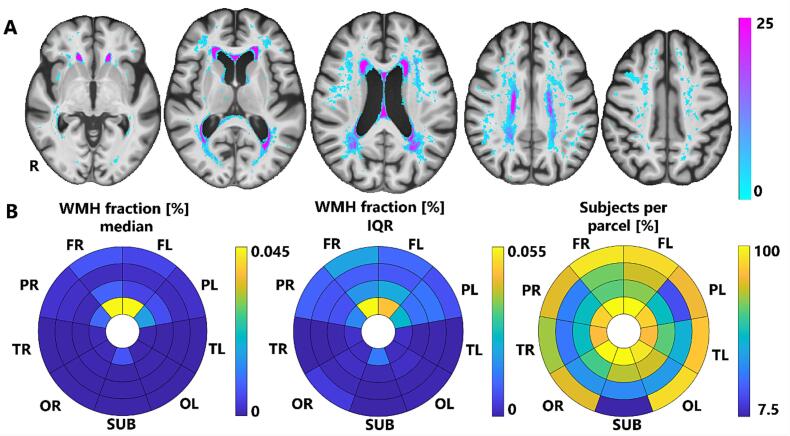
Spatial distribution of WMH. A. Spatial distribution of WMH at 7 T MRI. Fractions of subjects over the entire sample (%, N = 40) are represented. The resulting map is overlaid onto the MIITRA elderly template (axial slices are Z = 2, 13, 24, 34, 43, from left to right). B. WMH fraction distribution (normalized over ICV) indicated by the median (leftmost) and IQR (middle) values, respectively. The rightmost bullseye plot depicts how many subjects (as a fraction of the whole sample, %) showed a WMH lesion in each of the bullseyes’ parcels.
